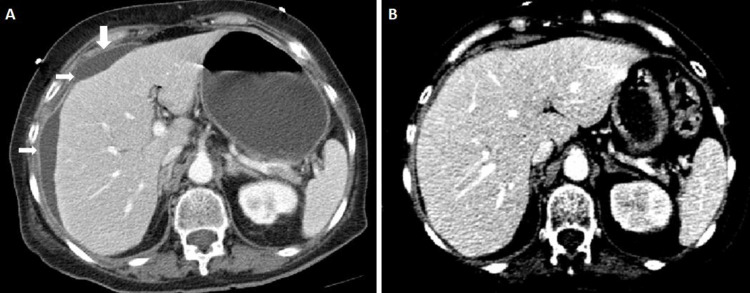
Contrast-Enhanced CT Scan Abdomen. (A) An axial selected section of the CT abdomen showing a hypodense fluid density collection in the subcapsular and perihepatic region of the liver, mainly in the right lobe (white arrows). (B) An axial selected section, from a follow-up CT scan abdomen showing the resolution of the previously mentioned collection. CT - computed tomography
